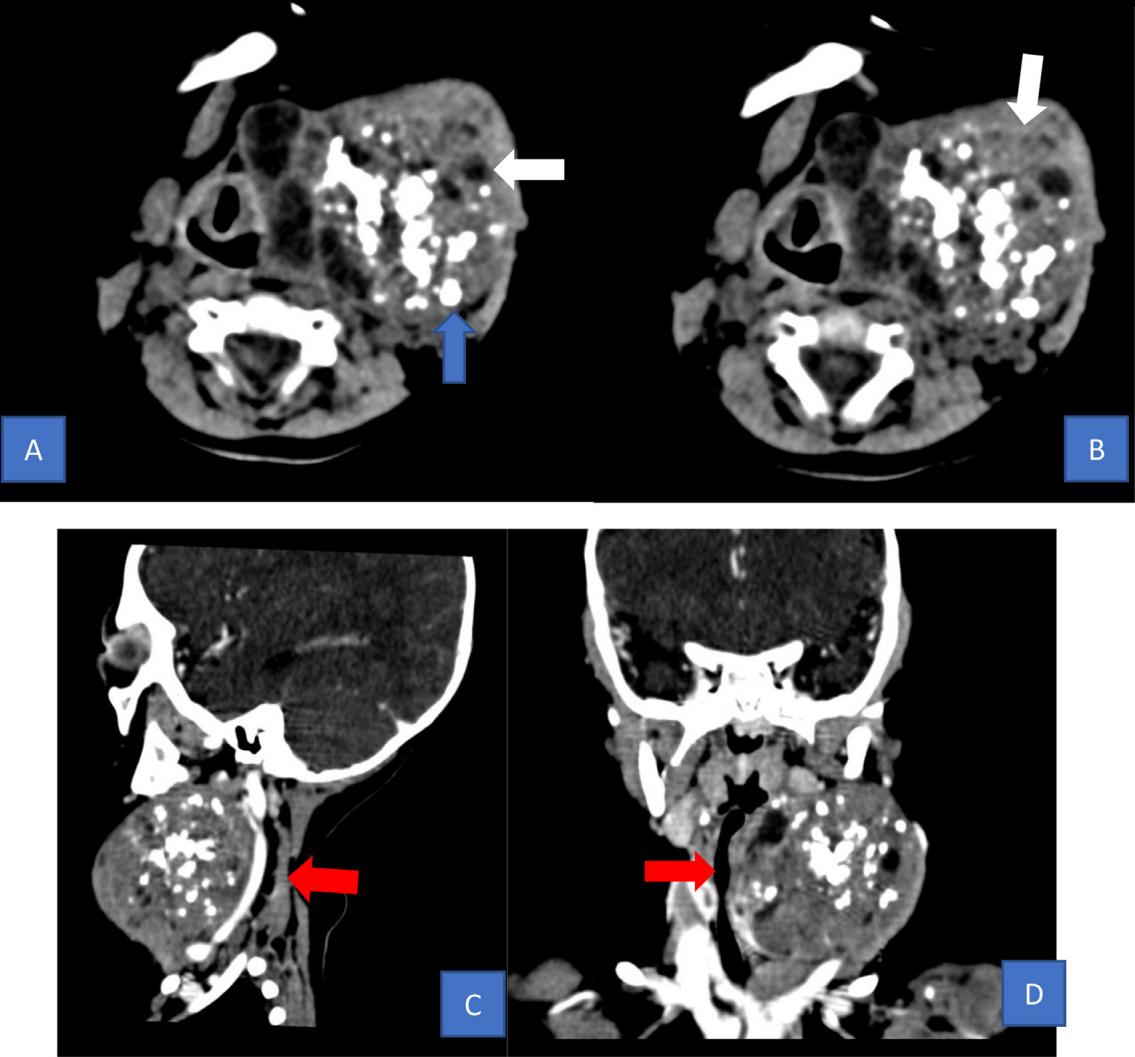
(A) CT scan of the left colli showed that there was a heterogeneous solid and cystic mass (white arrow) with lobulated borders that had calcifications (blue arrow). (B) An axial intravenous contrast-enhanced image showed no obvious enhancement. (C) The airway was neither compressed nor distorted (red arrow). (D) Coronal views in computed tomography.
